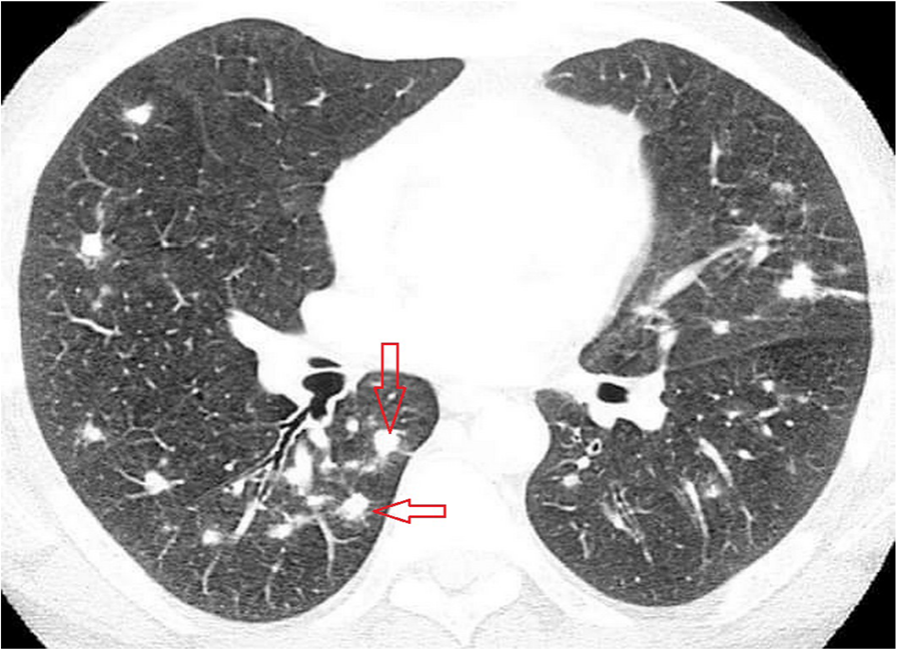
CT scan shows multiple irregular nodules in right middle and lower lobe, lingula and anterior segment of left lower lobe with solid nodules surrounded by a ground glass (red arrow)
